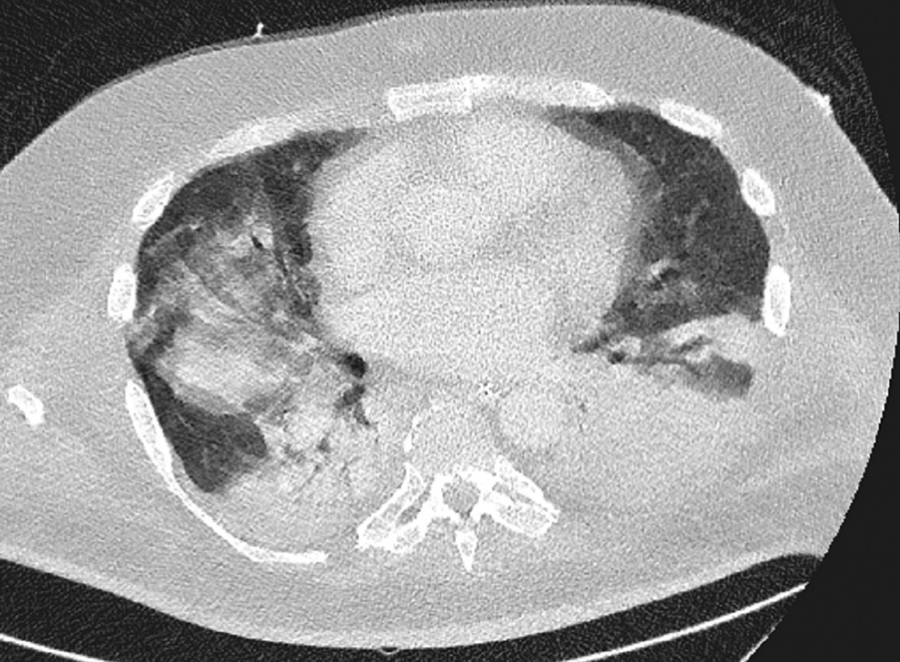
Transversal thoracic CT scan obtained on day 37 of ICU admission, revealing progression of the dense pulmonary lesion dorsal in the left lower lobe.
